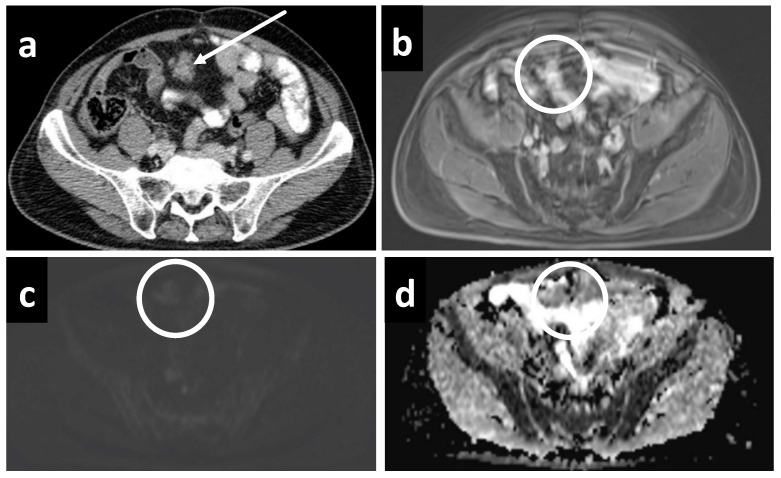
A fairly large soft tissue density peritoneal deposit is well seen on (a) CT, as it is surrounded by hypodense fat. Despite its large size, it is obscured on MRI with (b) delayed, (c) DWI, and (d) ADC sequences, primarily due to motion artifacts.
